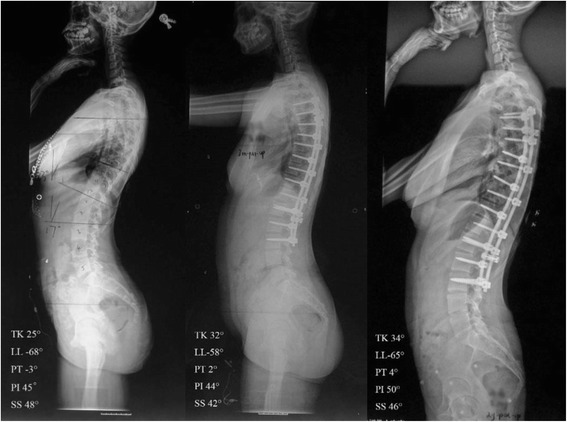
This 14-year-old girl presented with adolescent idiopathic scoliosis. The main thoracic Cobb angle was 41°. The pre-, post-operative and 2-year follow-up sagittal parameters were shown in the radiograph. The oTK calculated from the formula were 28.68°, 25.89° and 28.01° respectively
